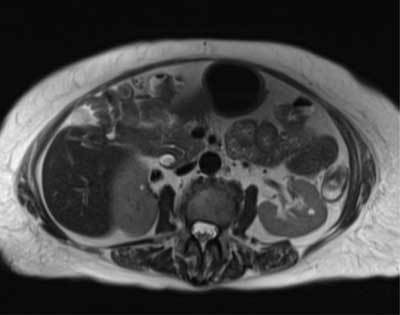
Axial T2-weighted images show a possible communication between the gallbladder and duodenum/colon (white arrow).
